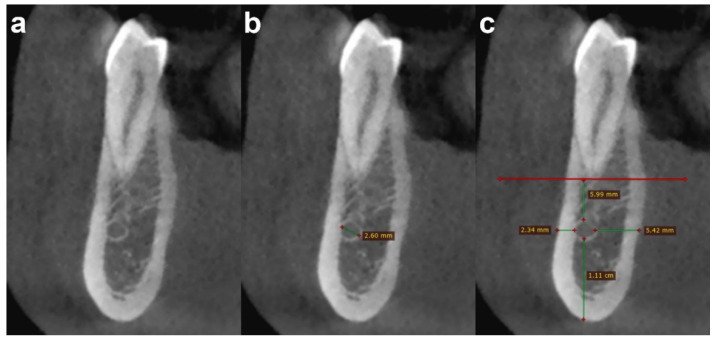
Cross-sectional CBCT images show an example of the steps of image analysis of the MIC at the first premolar level, as follows: (a) detection; (b) diameter measurement; and (c) distance measurement from adjacent anatomical landmarks (cortical plates and first premolar apex).
